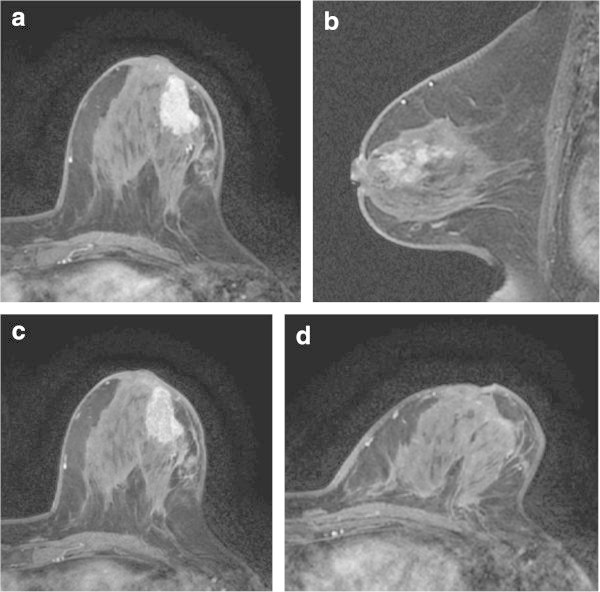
HER2 breast cancer of left breast in 71-year-old woman. Prior to NAC, dynamic MR images obtained at 60 s (a), 240 s (b), and 300 s (c) after administration of gadolinium show a strong and washout enhancing mass with a smooth border. Tumor extension around the mass is shown in Figure 2b. After the completion of NAC, there is no longer recognizable tumor on dynamic MR image obtained at 300 s (d).
